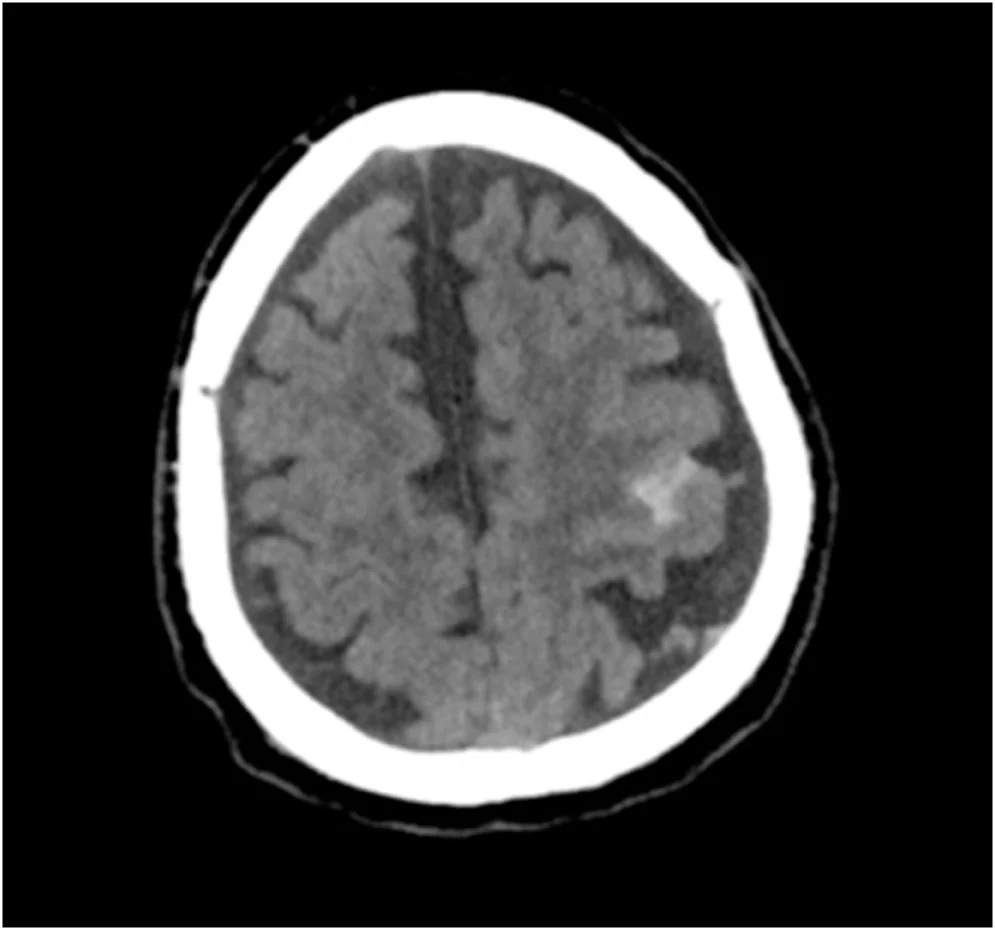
CT images of focal SAH at initial admission.
